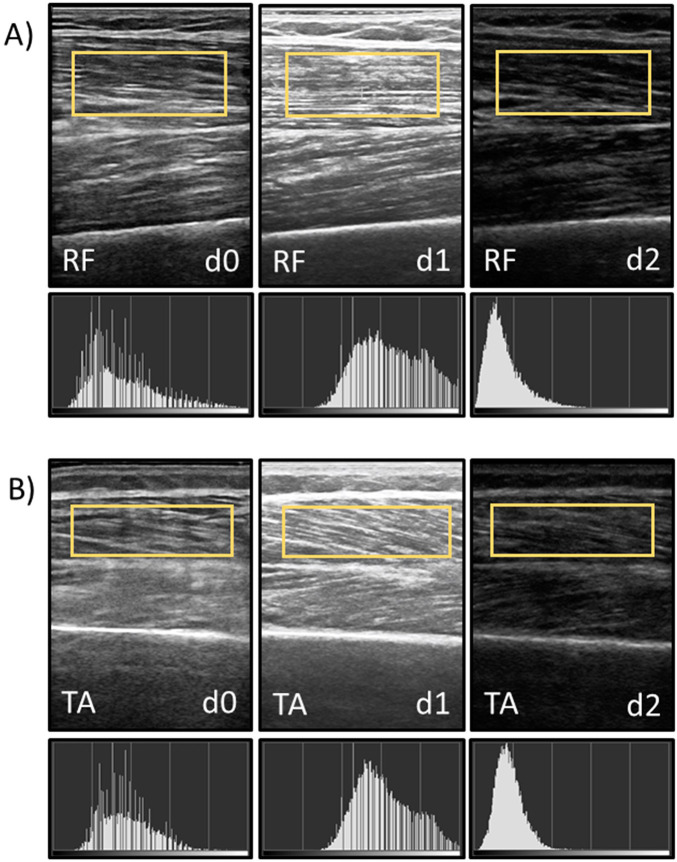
Exemplar longitudinal ultrasound images (top) and corresponding grayscale histograms (bottom) of the (A) rectus femoris (RF) and (B) tibialis anterior (TA) muscles from a single participant, acquired with three ultrasound devices (d0: hitachi-aloka noblus, d1: philips EPIQ 7 180 plus, d2: sonoSite titan). Histograms were generated from the inscribed rectangular regions of interest. Histogram x-axes represent grayscale intensity values from 0 to 255, and y-axes represent relative pixel frequency within the region of interest. B-mode images are shown as qualitative exemplars and are not presented with a common spatial scale.
